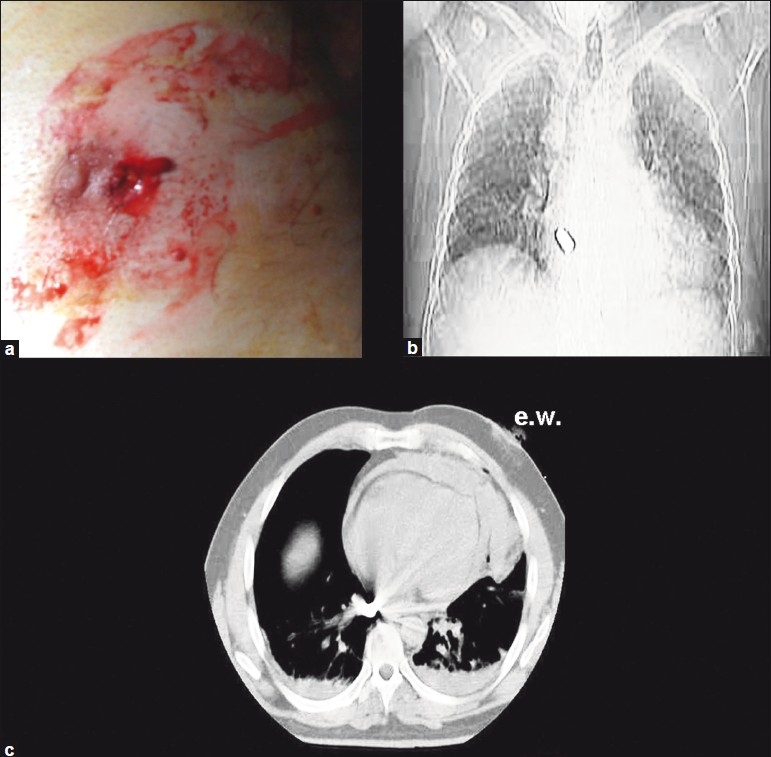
a) The bullets left nipple line wound; b) chest X-ray shows the intracardiac bullet; c) CT of the thorax reveals bilateral hemothorax and a huge fluid collection in the pericardium. The ribs were undamaged. The trajectory of the bullet was deviated by the finger seems to be from the left to the right side of chest
